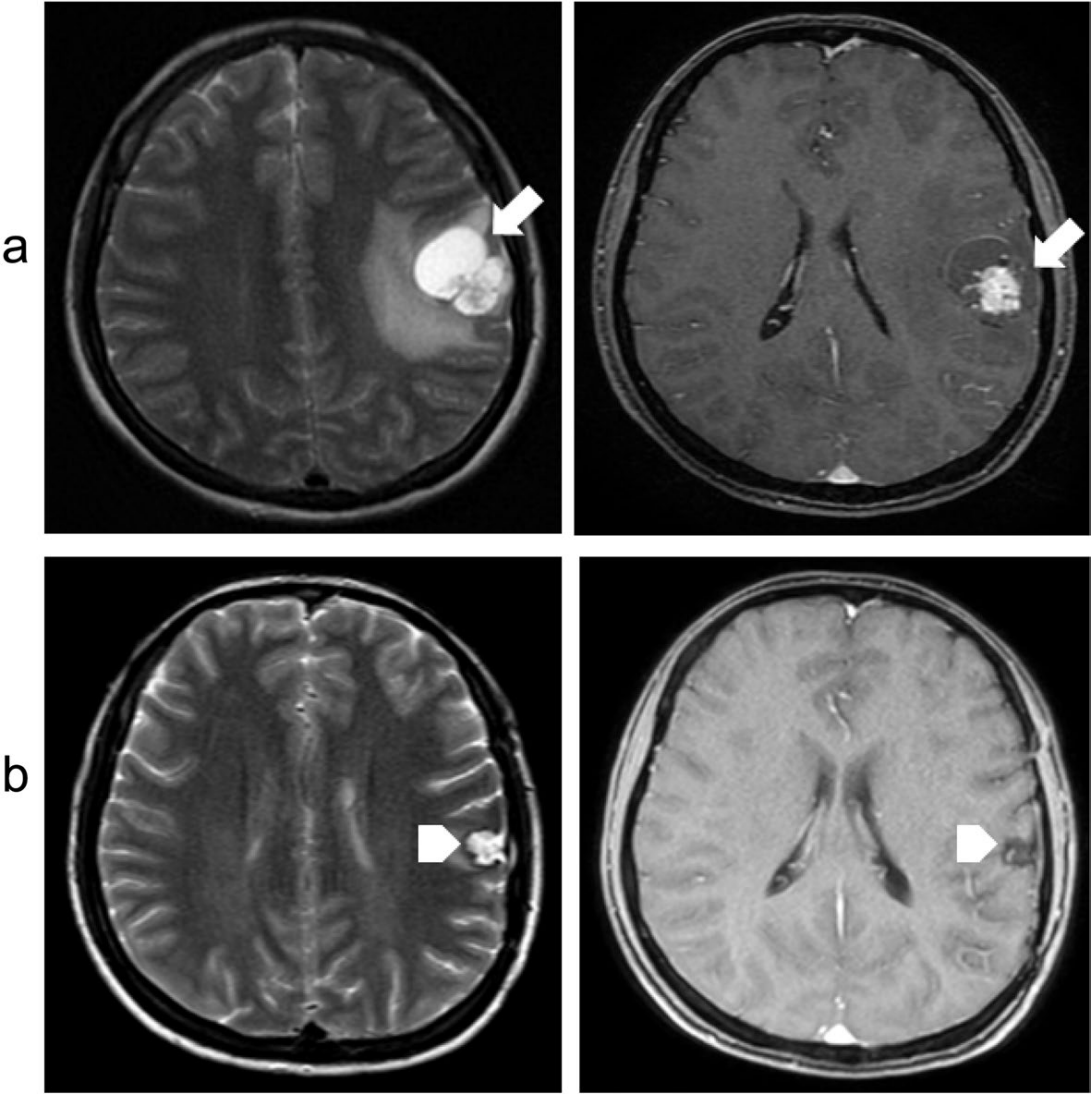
Pre- and post-surgery MRI shows lesions in the left temporal region (a): Pre-surgery MRI showed that a cystic portion showed high intensity on T2-weighted and a solid portion showed contrast enhancement on T1 -weighted in the left temporal, and extensive cerebral edema around the tumor (arrow-upper row) (b): Three months post-surgery MRI (lower row) shows a lesion with high signal intensity on T2 weighted, low signal intensity without post-contrast enhancement on T1 weighted (arrowheads)
